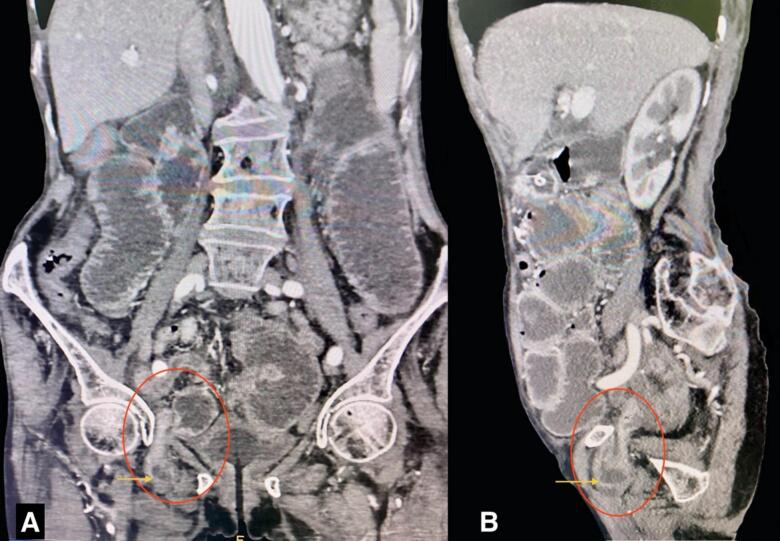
CECT coronal(A) and sagittal(B) section showing right-sided obturator hernia with small bowel (yellow arrow) herniating through the right obturator canal. CECT: Contrast-enhanced computed tomography. (For interpretation of the references to colour in this figure legend, the reader is referred to the web version of this article.)
